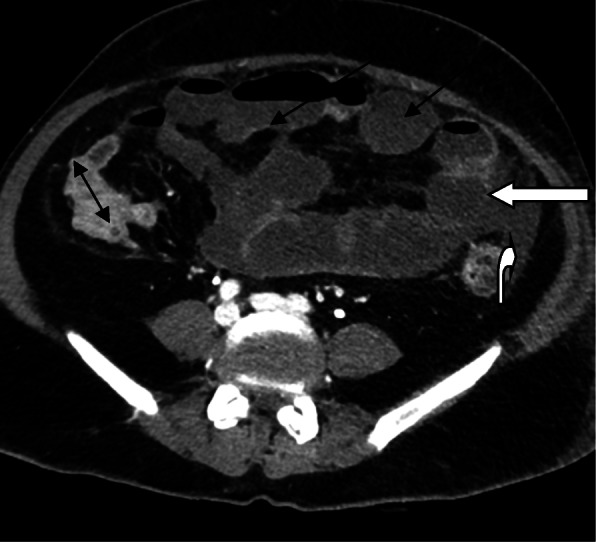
Axial post-contrast CT abdomen done for ICU patient with abdominal distention with limited sonographic assessment with distended small bowel loops (long black arrows), collapsed large bowel loops (double-headed black arrow), there is small bowel segmental mural poor enhancement is noted (straight white open arrow) with related mild collection (curved white open arrow)
